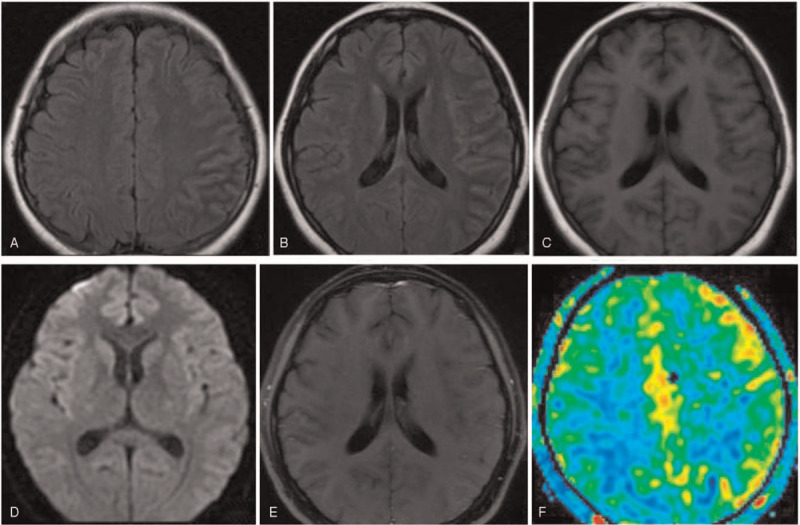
Brain MRI of our patient with anti-MOG-associated cerebral cortical encephalitis. On admission, axial T2-FLAIR images showed hyperintensity and swelling of the left cerebral cortex (A, B), without signal intensity changes on T1-weighted and diffusion-weighted images (C, D). Axial T1-weighted image post-gadolinium revealed no contrast enhancement (E). ASL image showed focal hyper-perfused areas involving the left cerebral cortex (F). ASL = arterial spin labelling, MRI = magnetic resonance imaging, T2-FLAIR = T2-weighted fluid-attenuated inversion recovery.
